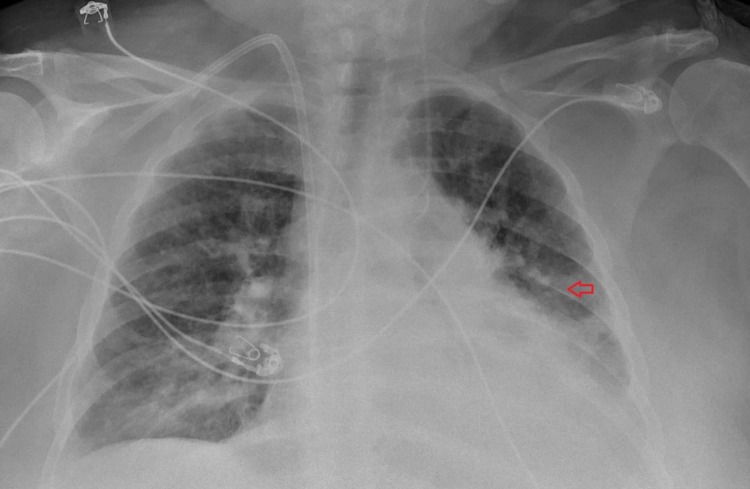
Repeat chest x-ray after pericardial drainage showing improved aeration of the lung (red arrow)
